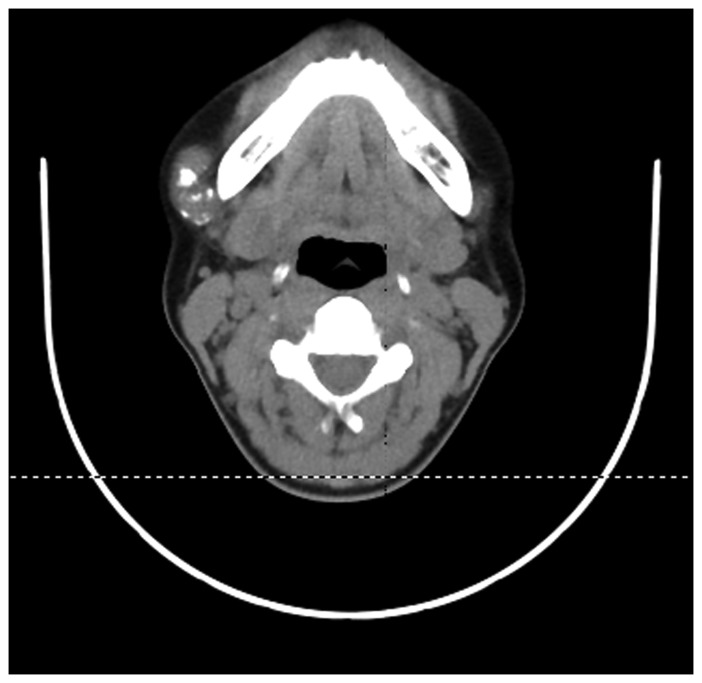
Computed tomography scan revealing a soft-tissue mass with dense calcification located in the right buccal region.
